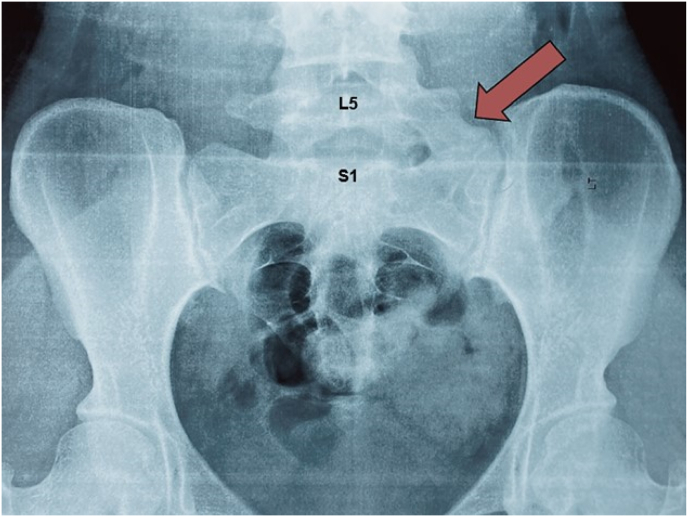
Modified Anteroposterior pelvis radiograph of the patient with central X-rays pointing towards the anterior end of the body focusing along the midline 5 cm directly below the anterior superior iliac spine, also known as Ferguson radiograph. A red arrow demonstrates abnormal overgrowth of the transverse process emerging from the L5 segment and its complete fusion with the left sacral ala consistent with type IIIA LSTV.
